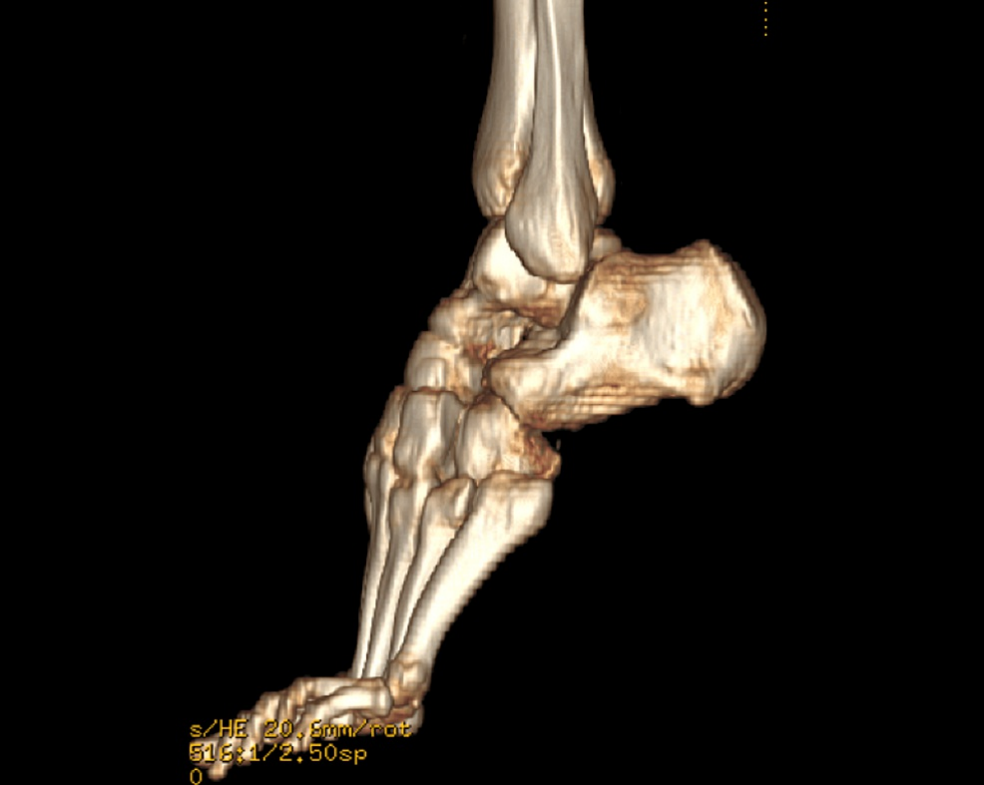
Three-dimensional CT view of the foot pre-operatively demonstrating the dislocation of the cuboid.
